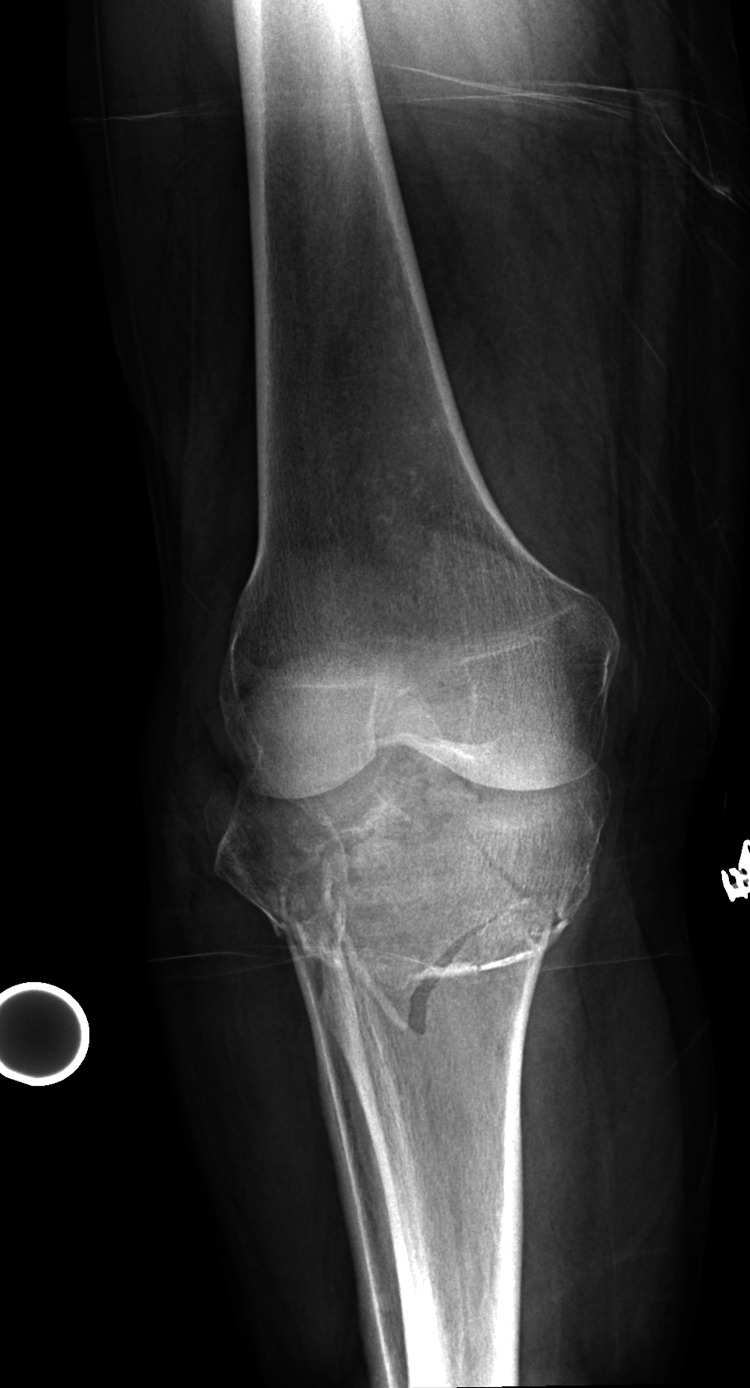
AP radiograph of the injured limb. The fracture is a comminuted but impacted proximal tibia fracture.
